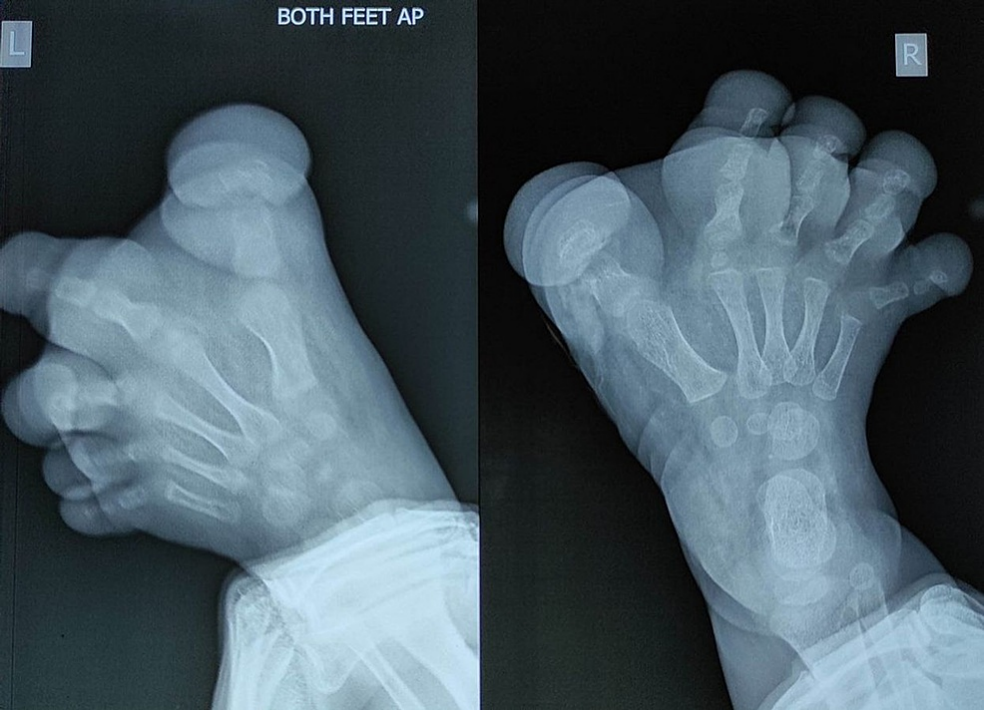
X-ray AP view of bilateral feet showing bony hypertrophy and overgrowth of all the bones with increased soft tissue shadow. AP: anteroposterior, L: left, R: right.
